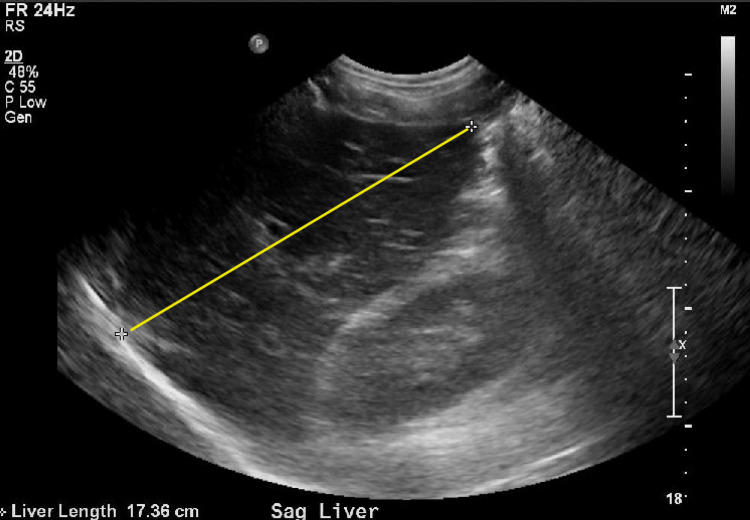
Right upper quadrant ultrasound of the abdomen shows a liver span of 17.36 cm
